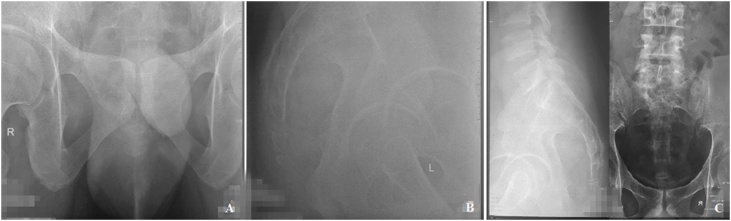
Pelvis and Lumbosacral Plain Radiograph of the Patient.
